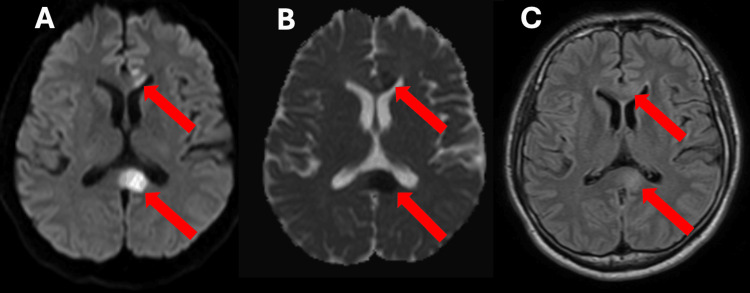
Cranial MRI findings (A) Diffusion-weighted imaging (DWI) shows areas of hyperintensity involving the body and splenium of the corpus callosum (red arrows), consistent with restricted diffusion. (B) Apparent diffusion coefficient (ADC) map demonstrates corresponding hypointense signals in the same regions (red arrows), demonstrating true diffusion restriction. (C) Fluid-attenuated inversion recovery (FLAIR) sequence shows subtle hyperintensity in the body and splenium of the corpus callosum (red arrows), corresponding to the areas of restricted diffusion.
